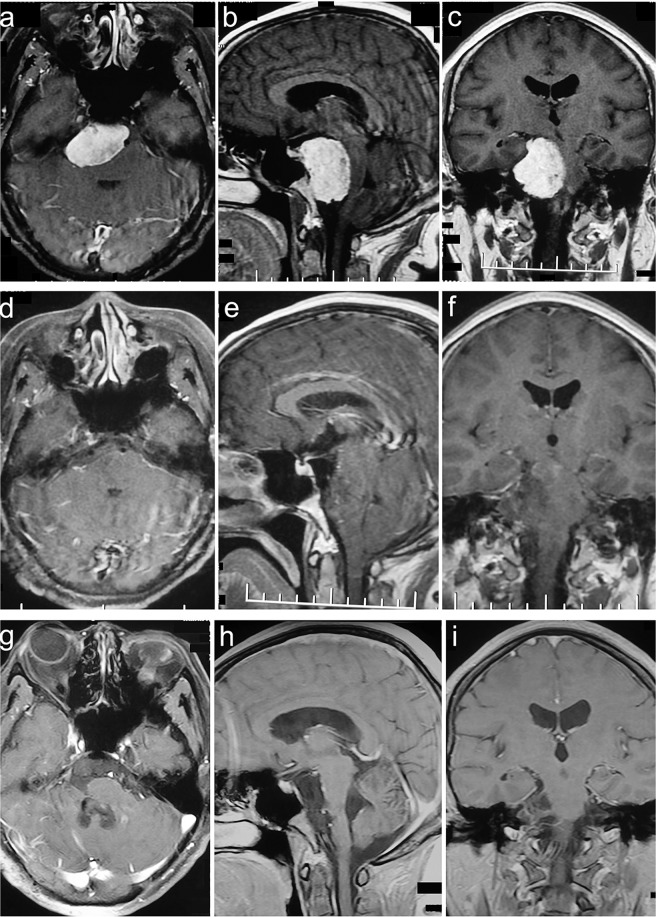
Clivus type case. A 56-year-old female presented with headache, dysphagia, bucking and hearing impairment for 84 months. The preoperative KPS score was 80. She was achieved GTR with the RSA of palsy in CN V and VII. The postoperative KPS score was 50. With a follow-up of 75 months, she participated in normal activities and had a KPS score of 90 without recurrence. (a–c) Preoperative MRI T1 contrast axial, sagittal, and coronal images. (d–f) Postoperative MRI T1 contrast axial, sagittal, and coronal images. (g–i) Follow-up MRI T1 contrast axial, sagittal, and coronal images.
